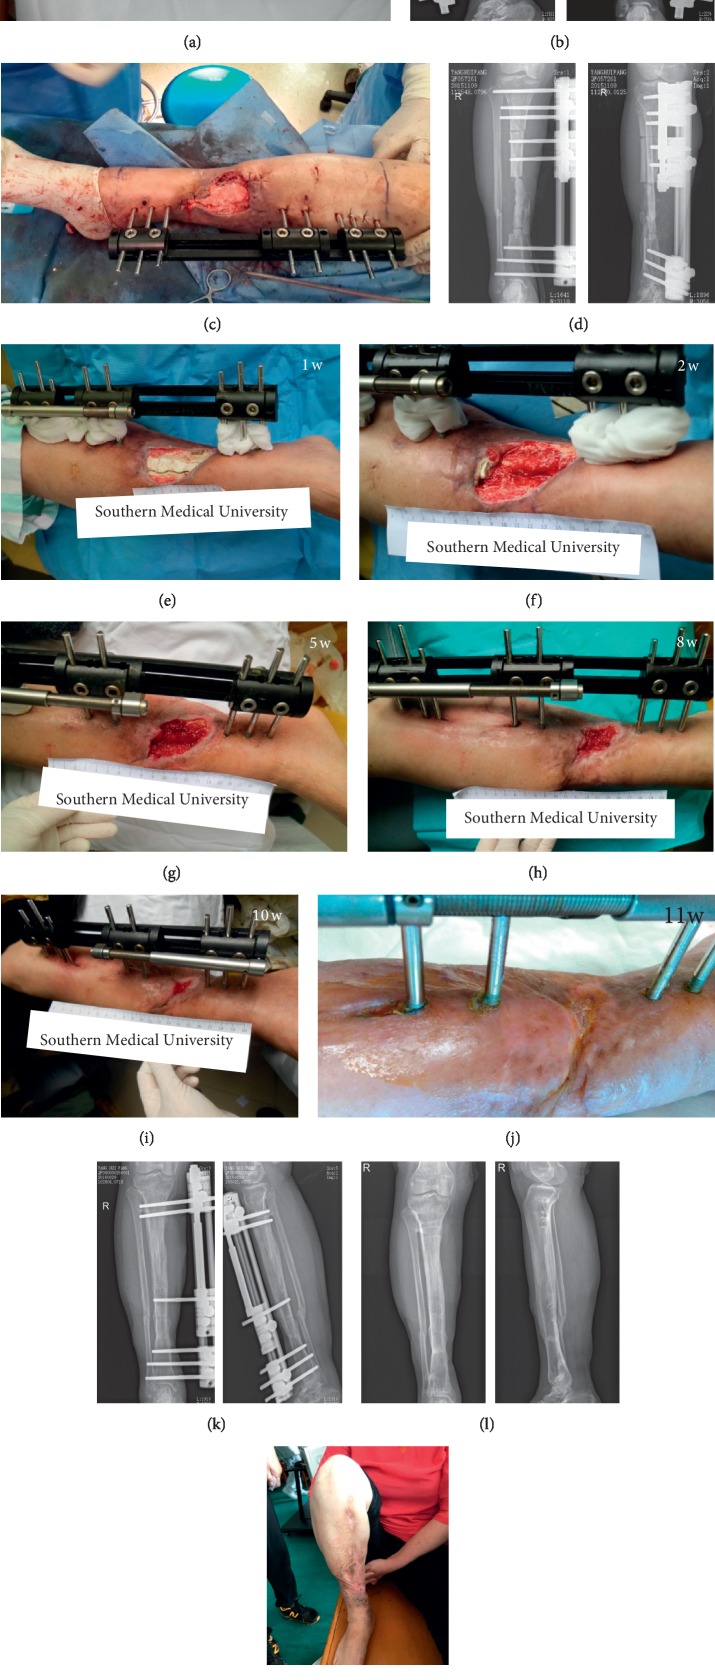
(a) The appearance of the affected limb at transfer to our department; (b) X-ray of tibia and fibula; (c) after debridement and replacement of the external fixator, the bone defect site was filled with a calcium sulfate spacer loaded with vancomycin, exposing the wound surface; (d) postoperative X-ray; (e–j) the appearance of the affected limb after operation. The wound surface gradually decreased and healed throughout the bone transport process; (k) postoperative X-ray at 11 months showed that the fracture was well healed and the external fixator was removed; (l-m) both the X-ray and the appearance at 24 months after the operation.
